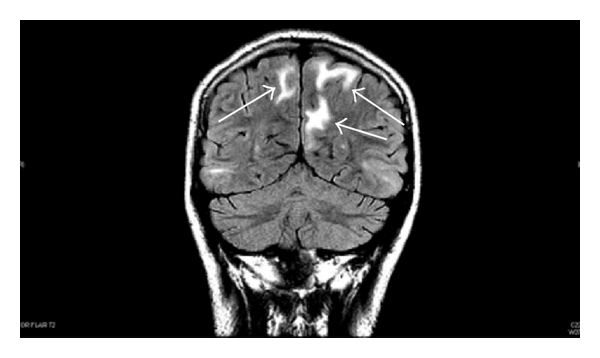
MRI T2 flair image demonstrating findings of posterior reversible encephalopathy syndrome involving bilateral parietal-occipital lobes, left greater than right (arrows).
